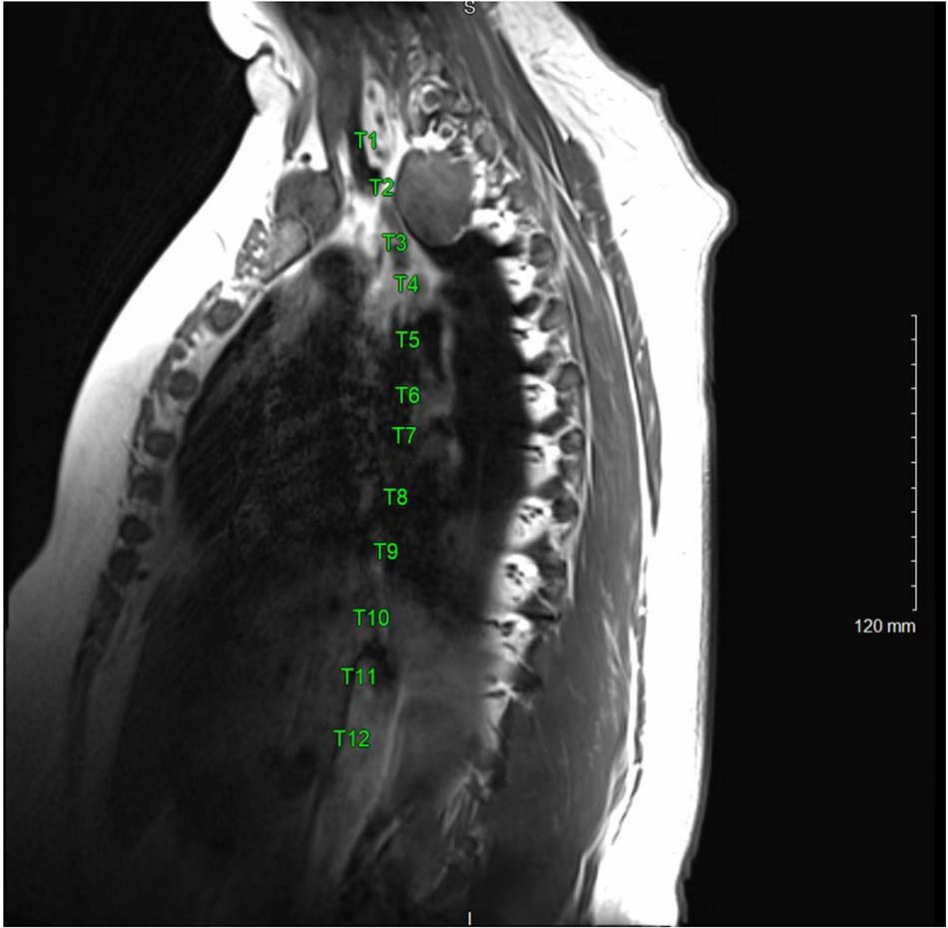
T1 MRI sagittal view of T2-T3 right paravertebral mass.
